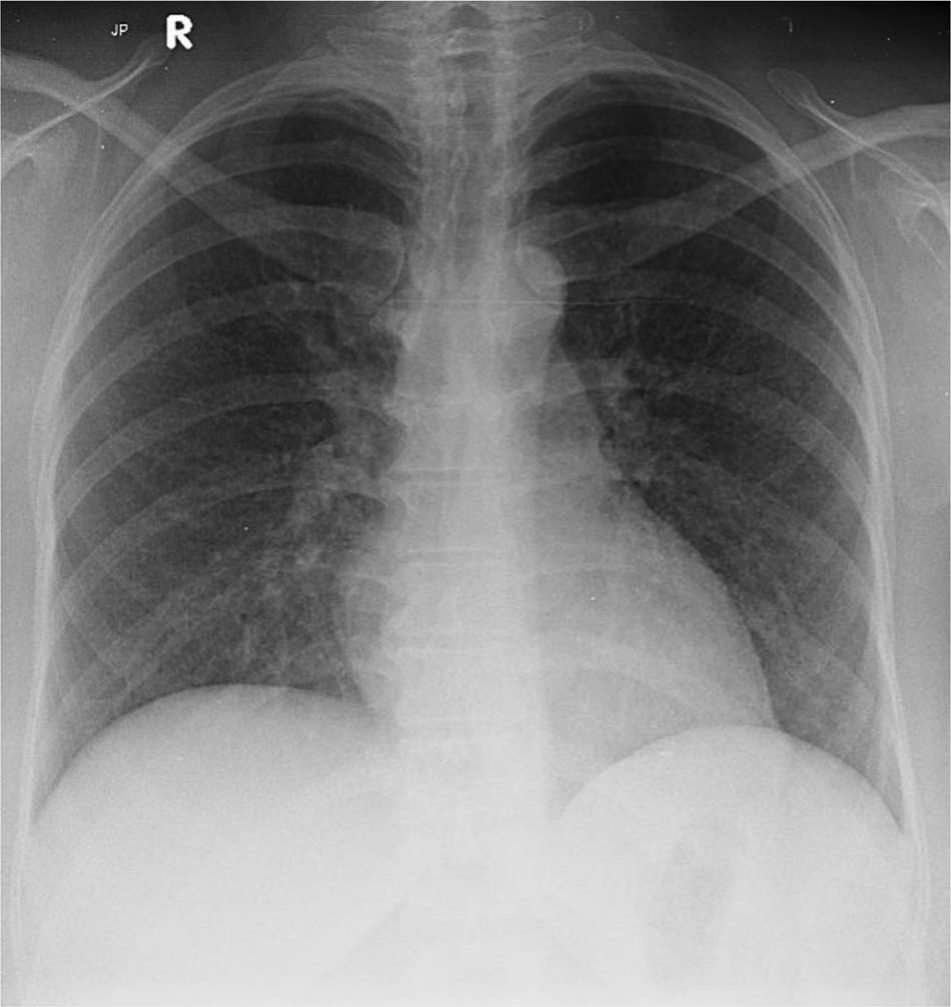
Chest radiograph showing diffuse bilateral reticulonodular opacities.
